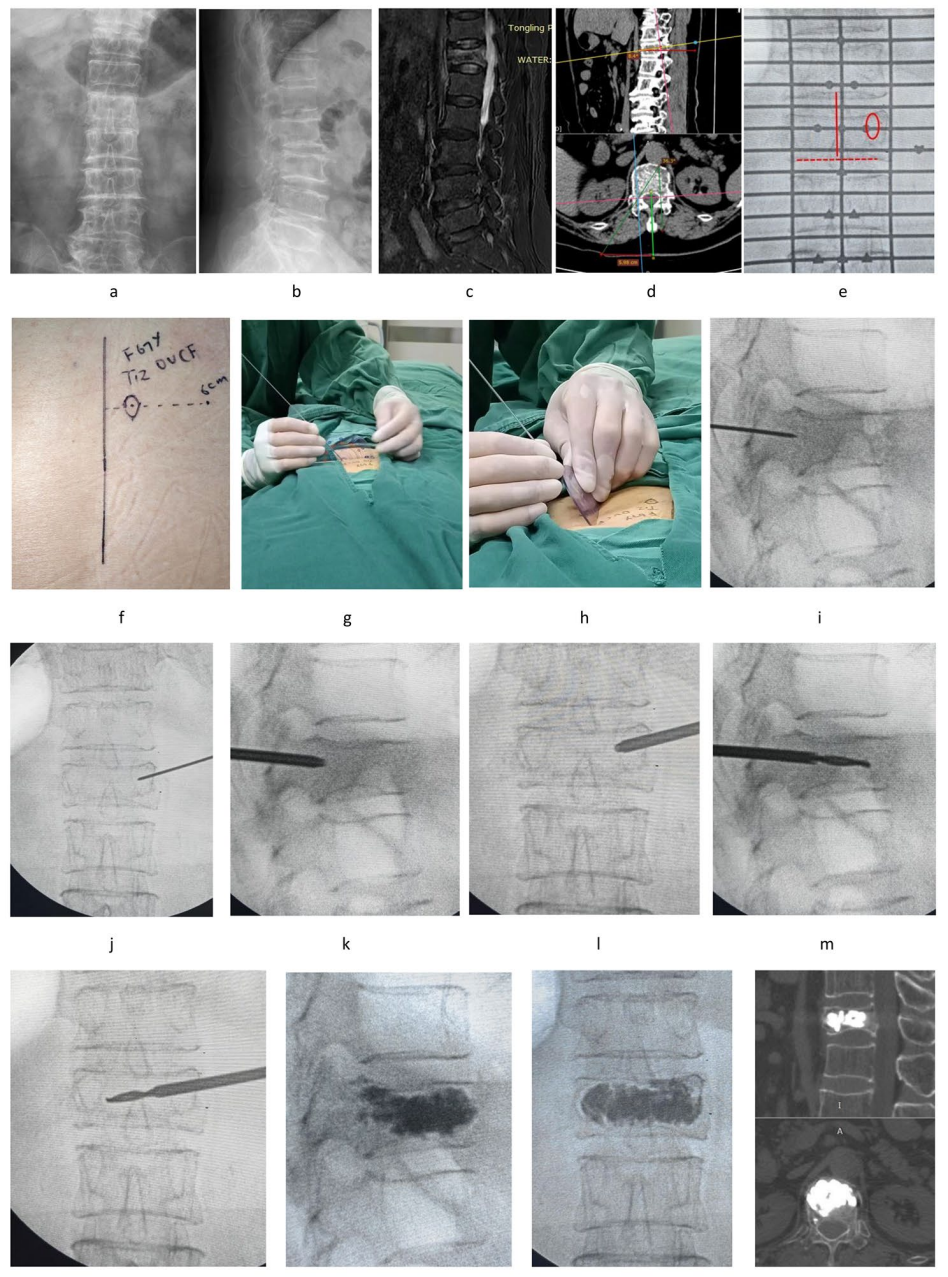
A 67-year-old female individual was diagnosed with OVCF at T12. a,b: Anteroposterior and lateral X-ray films before operation. c: A hyperintense intensity in the fractured vertebra was shown on the fat-suppressed MRI image before surgery. d: The puncture trajectory was designed through PACS. e, f: The puncture-side pedicle projection and the midline of the spine on the patient’s back skin were tagged. The puncture site was then confirmed according to Fig. 3d. Besides, the fractured vertebral inferior border (the red dotted line) does not have a double-layer projection. g, h: The abduction angle and sagittal inclination angle were adjusted by a protractor. i-n: The piercing process was shown. o-q: In the X-ray and 3D-CT pictures following surgery, the bone cement was effectively diffused in the fractured vertebra
